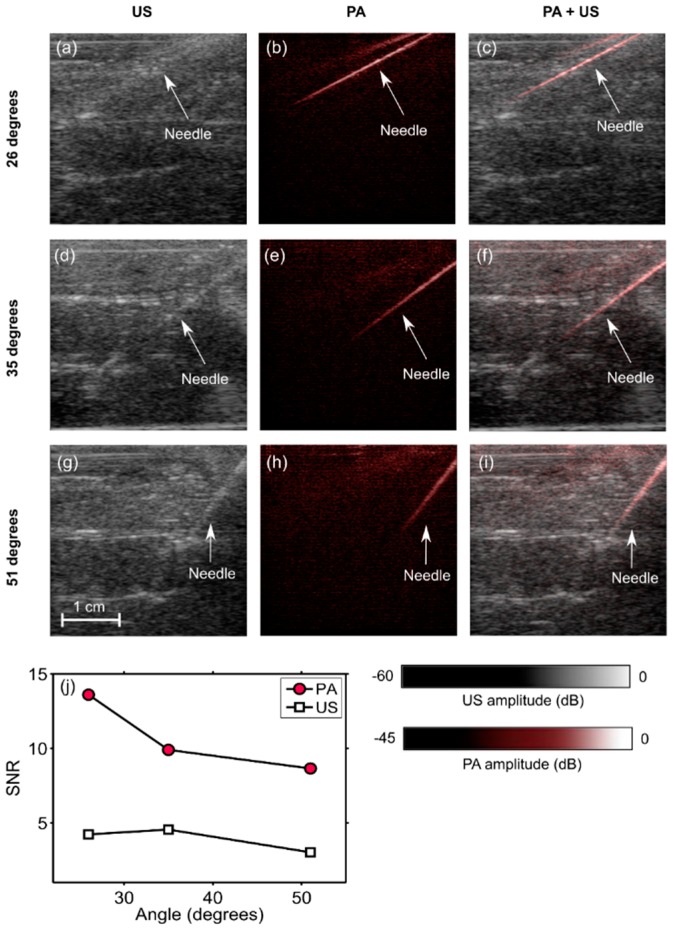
Photoacoustic (PA) images, ultrasound (US) images, and overlaid PA and US images (PA + US) of a spinal needle inserted into chicken breast tissue at different angles (a–i). Signal-to-noise (SNR) ratios for PA images were substantially higher than those for US images at all insertion angles (j). During the insertions, these images were reconstructed and displayed in real-time on a logarithmic scale. Here, they are presented without the uppermost 5 mm, which contained the ultrasound gel. Each point in the SNR plots was calculated from one spatial region.
